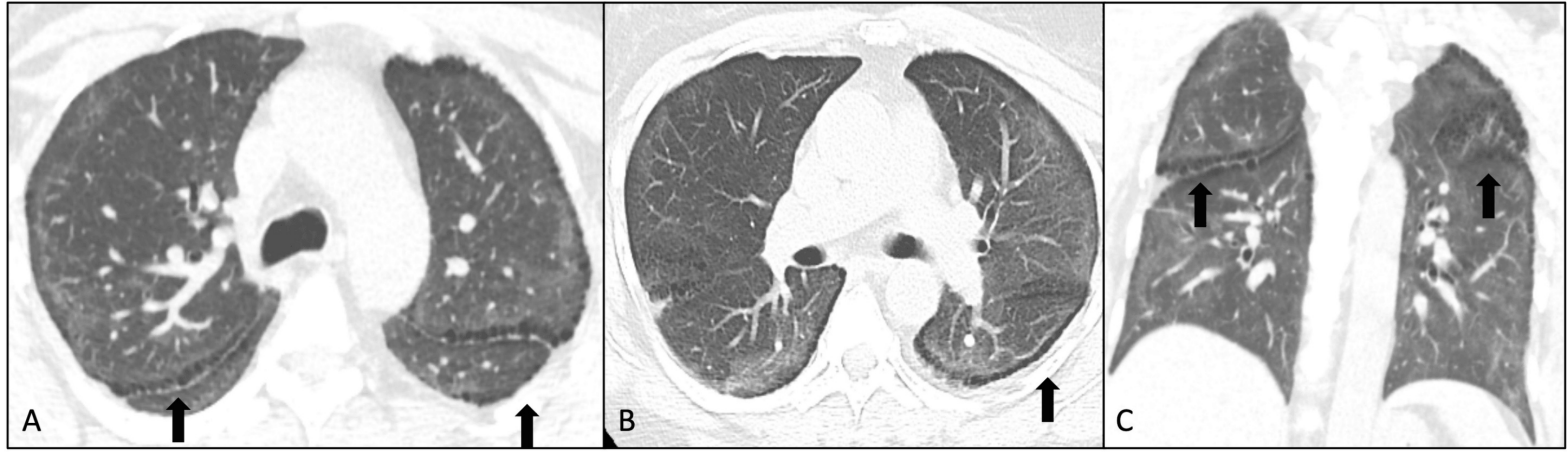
(A, B) High-resolution CT of the chest obtained four months after the initial scan demonstrates the resolution of consolidative opacities with persistent paraseptal emphysema-like lucencies (arrows). (C) CT of the chest shows similar findings along the pleura and the fissures (arrow).
